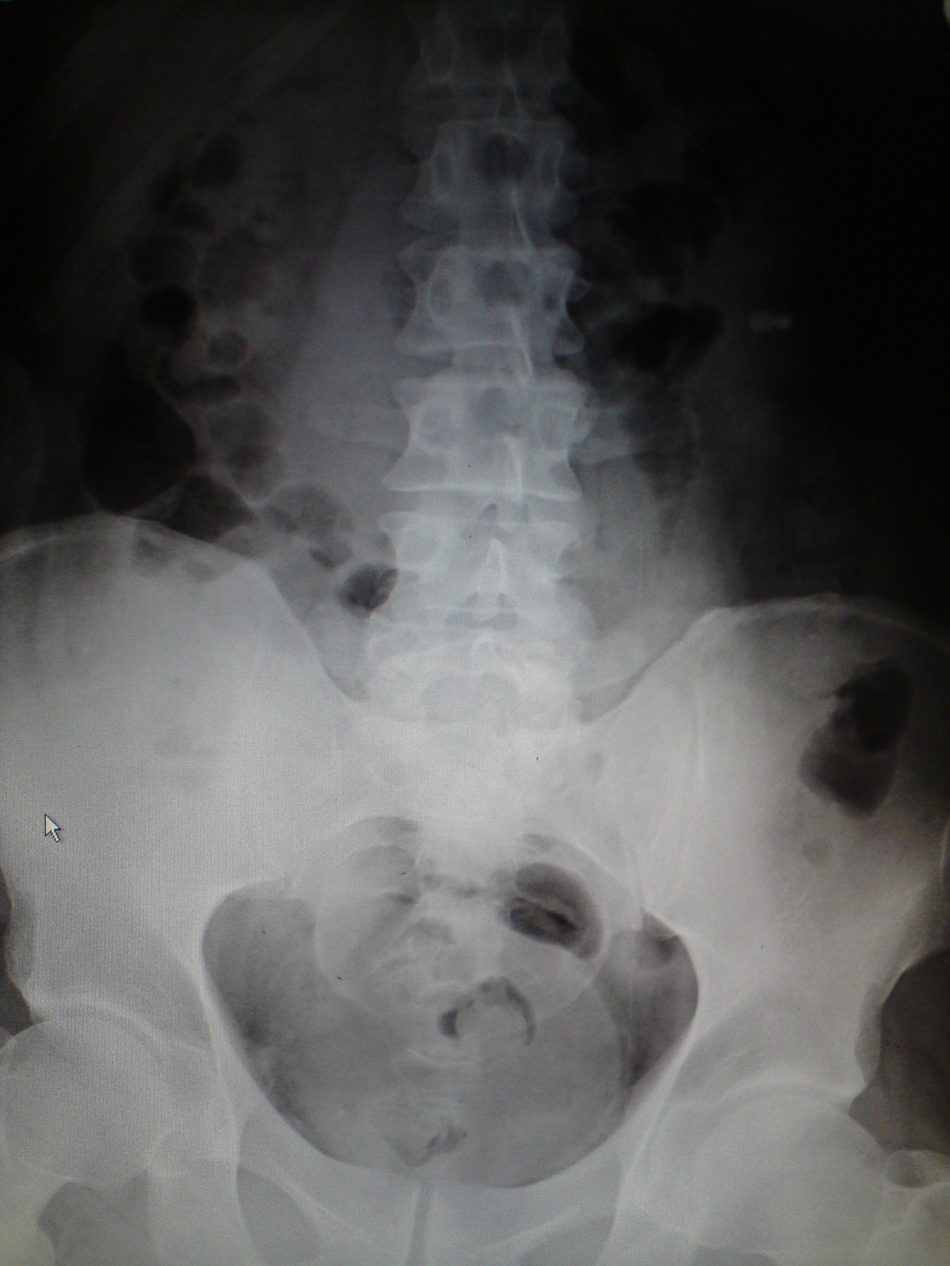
Normal plain film of the abdomen.
